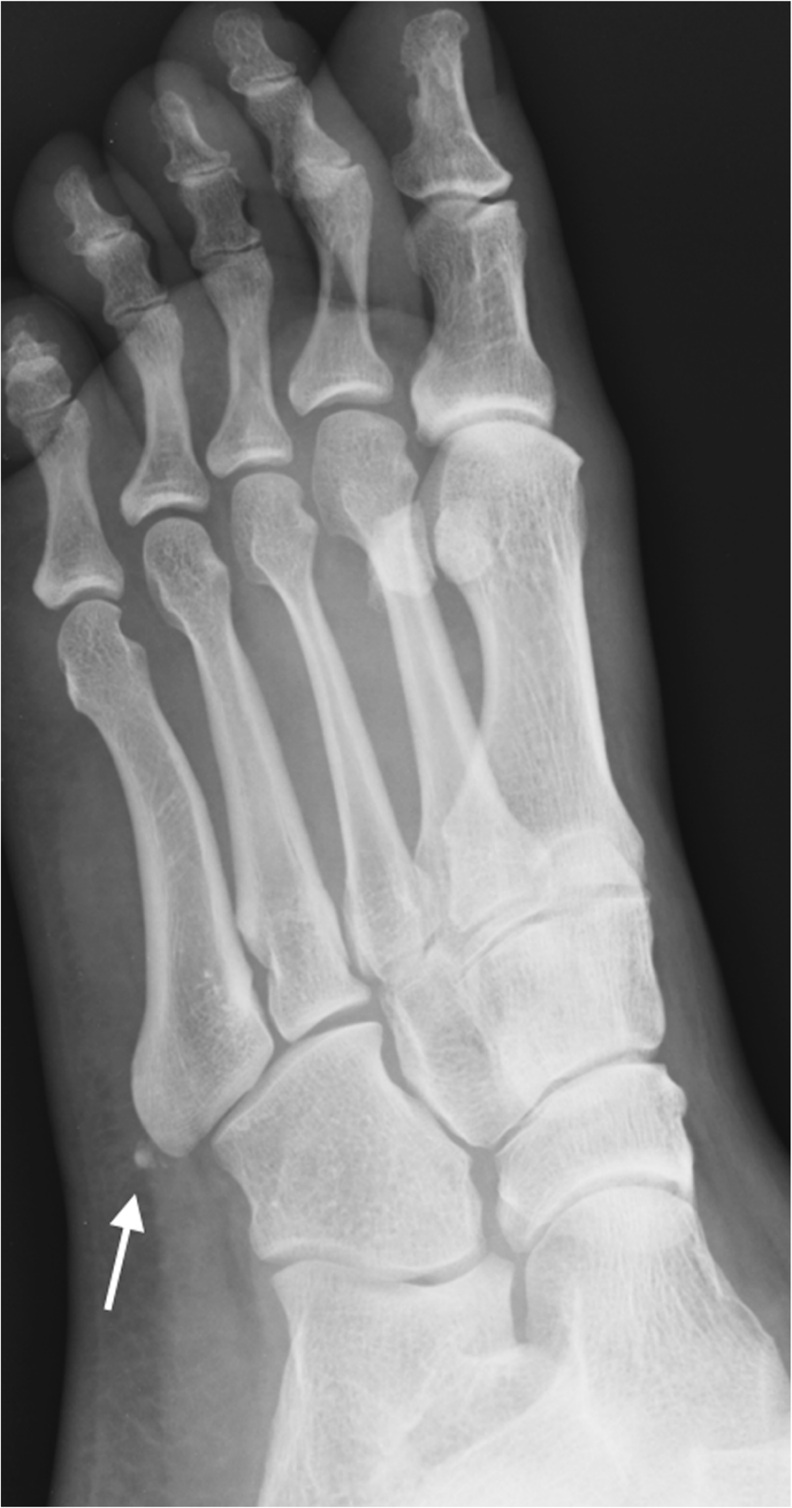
Os vesalianum. Oblique radiograph. Incidental finding of a small os vesalianum (white arrow). These very rarely constitute a source of pathology
